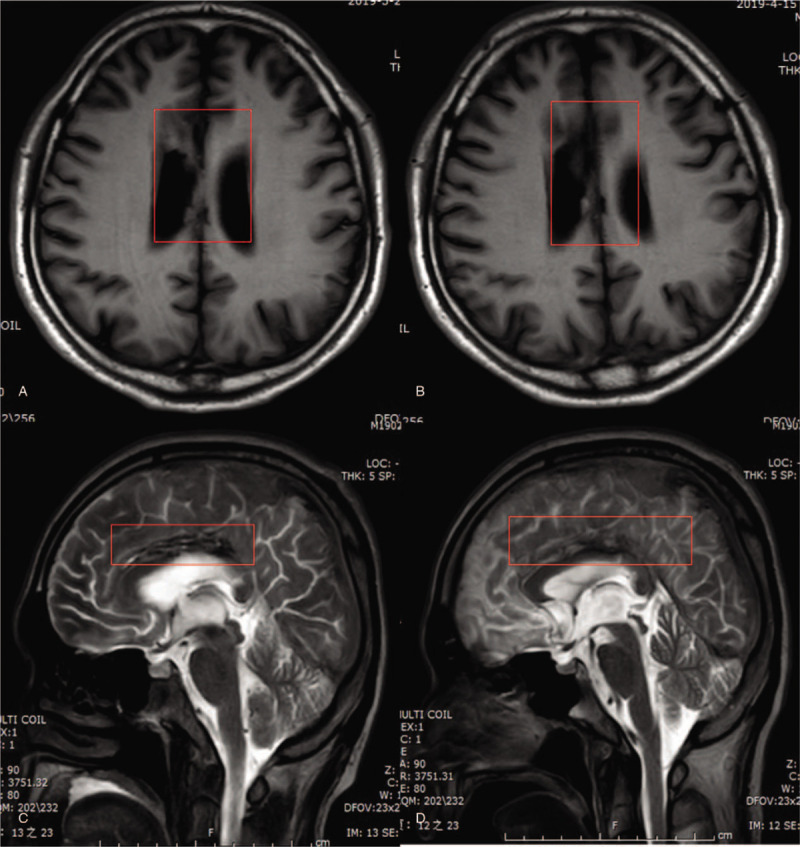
Conventional brain magnetic resonance imaging (MRI) changes. (A) and (C) show the MRI of the patient at admission. (B) and (D) show the MRI of the patient 1 month later.
